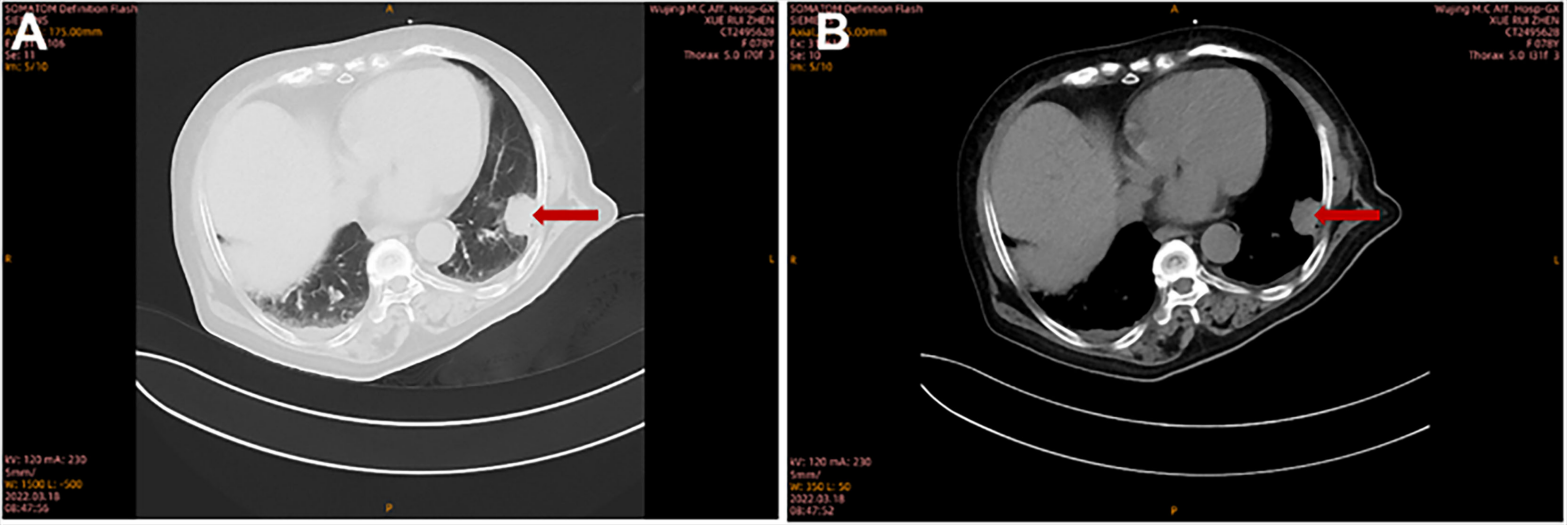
The imaging characteristics of PPP on pulmonary CT scan. Chest CT showed a solid lesion in the left lower lobe at lung window (A) and at mediastinal window (B).
